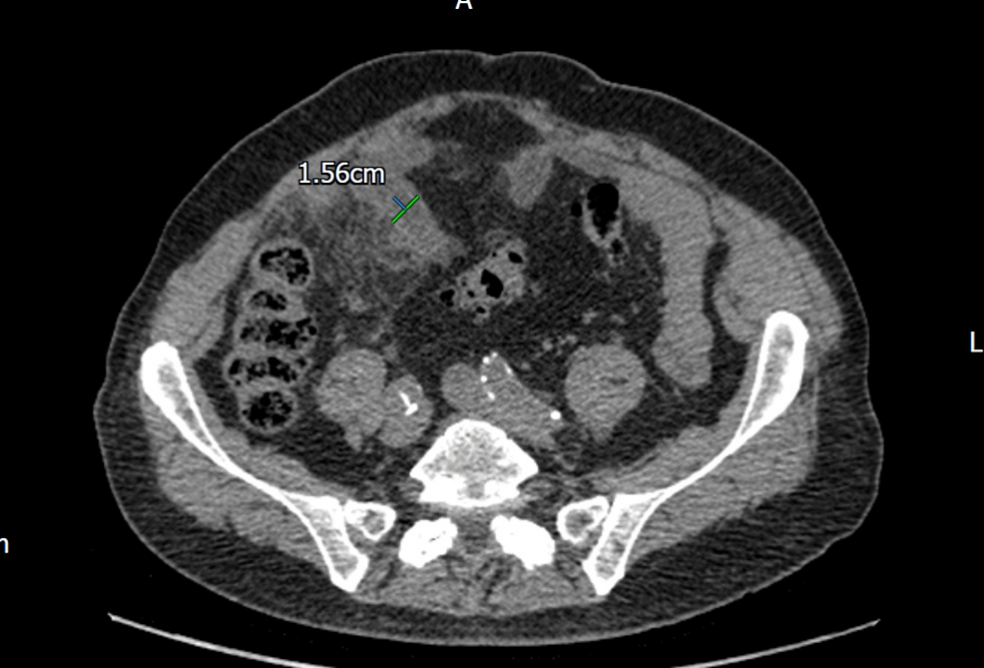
Axial CT scan - a highly suspicious ruptured appendix with acute appendicitis and focal changes.
